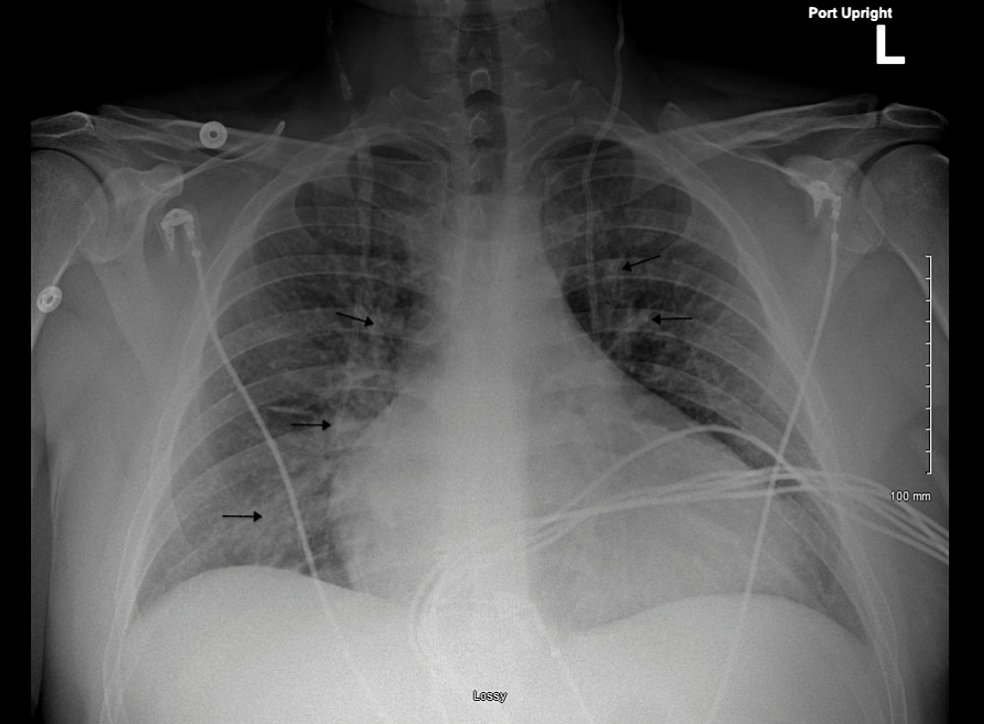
Chest x-ray showing interstitial pulmonary edema.
